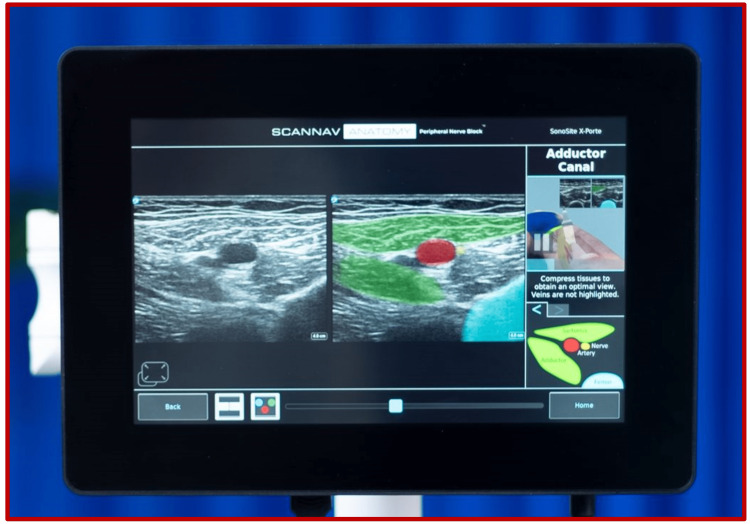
The ScanNav Anatomy Peripheral Nerve Block (Intelligent Ultrasound, Cardiff, UK) technology. Source: https://www.intelligentultrasound.com/wp-content/uploads/2022/10/DSC03437sq-1024x1024.jpg.
